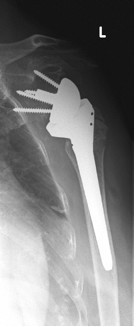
Antero-posterior radiograph of a 56 year-old male patient ´ s left shoulder with an implanted inverted total shoulder prosthesis Delta at 52 months of follow-up. Radiological analysis reveals "grade 2 = notch with condensation" of infraglenoidal scapular notching according to Nerot.
